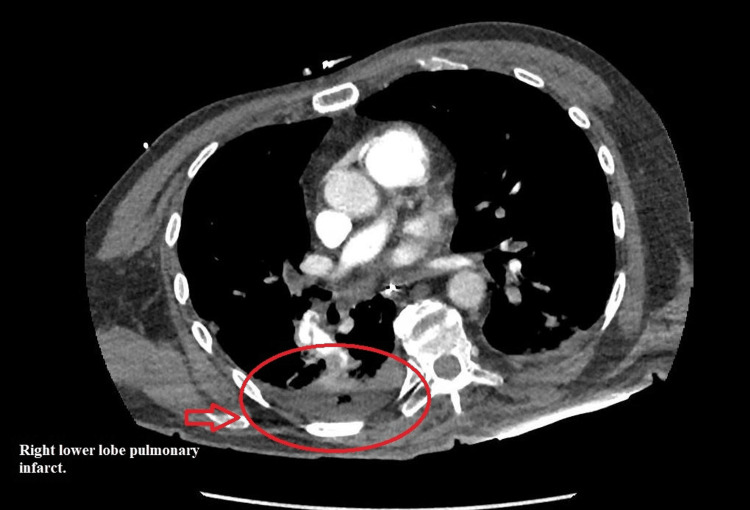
Computed tomography chest image demonstrating a peripheral wedge-shaped opacity in the right lung (arrow), representing pulmonary infarction secondary to distal pulmonary artery occlusion.
